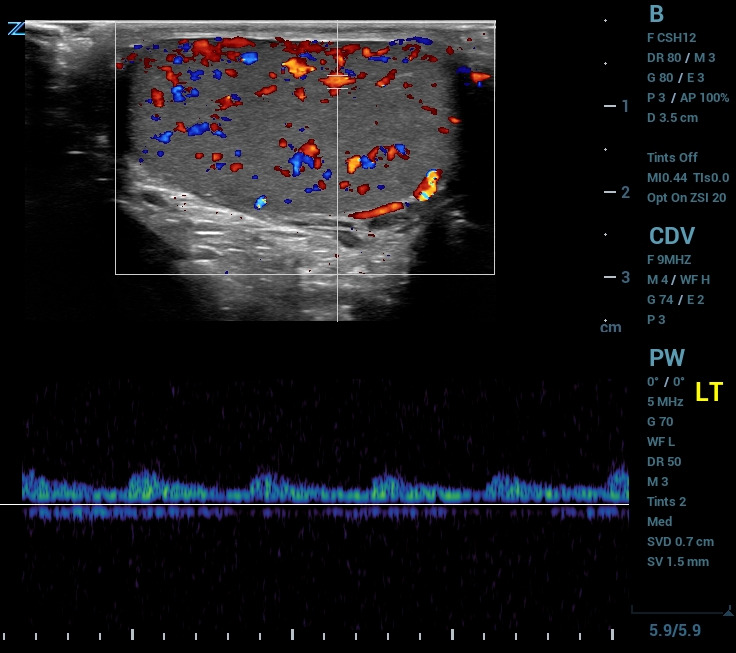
Spectral Doppler image of normal, low-resistance testicular arterial blood flow. Note the homogenous echogenicity and low velocity blood flow that consistently stays above the baseline.
